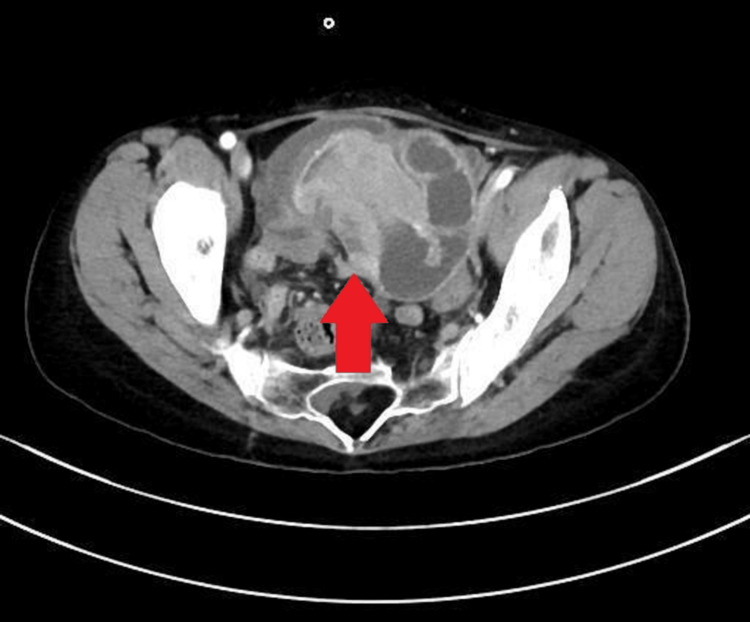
Contrast-Enhanced Computed Tomography (CT) at Relapse CT imaging demonstrates a newly developed small intestinal mass (arrow) causing small bowel obstruction after initial response to rituximab therapy.
